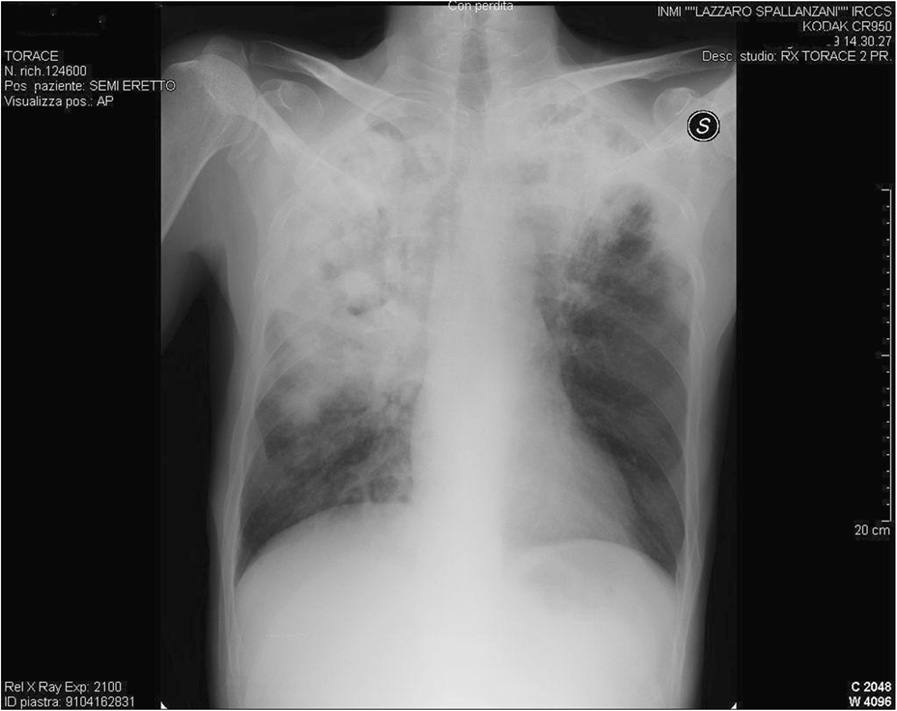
Posteroanterior chest radiography: extensive upper right subclavian and apex left subclavian infiltrates with cavitation.
